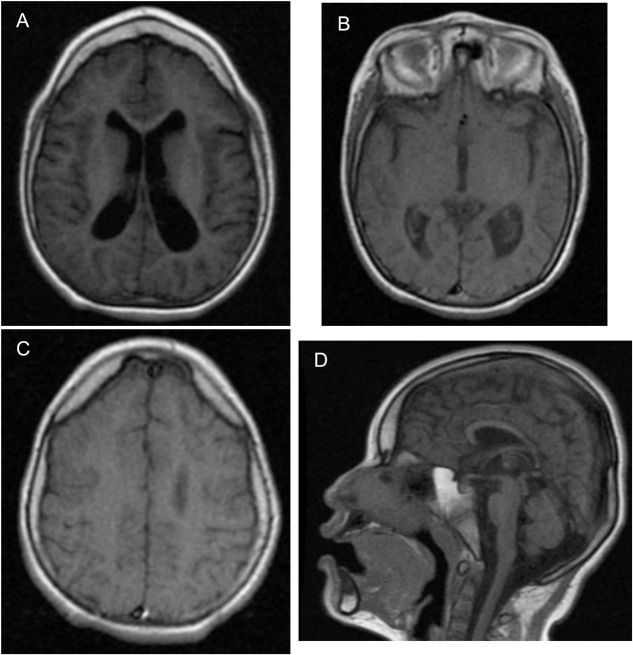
Abnormal brain MRI of the patient at 5 years old. (A) Cortical–subcortical frontotemporal atrophy, hypoplasia of the frontal lobes, and ventriculomegaly predominantly on the left on axial on axial T1-weighted FLAIR MR image. (B) Enlarged Sylvian cistern and ventriculomegaly predominantly on the left on axial T1-weighted MR image. (C) Frontotemporal cortical dysplasia pachygyria and polymicrogyria on axial T1-weighted MR image. (D) Hypoplastic *corpus callosum* and enlargement of *cisterna magna* without cerebellar atrophy on sagittal T1-weighted MR image.
